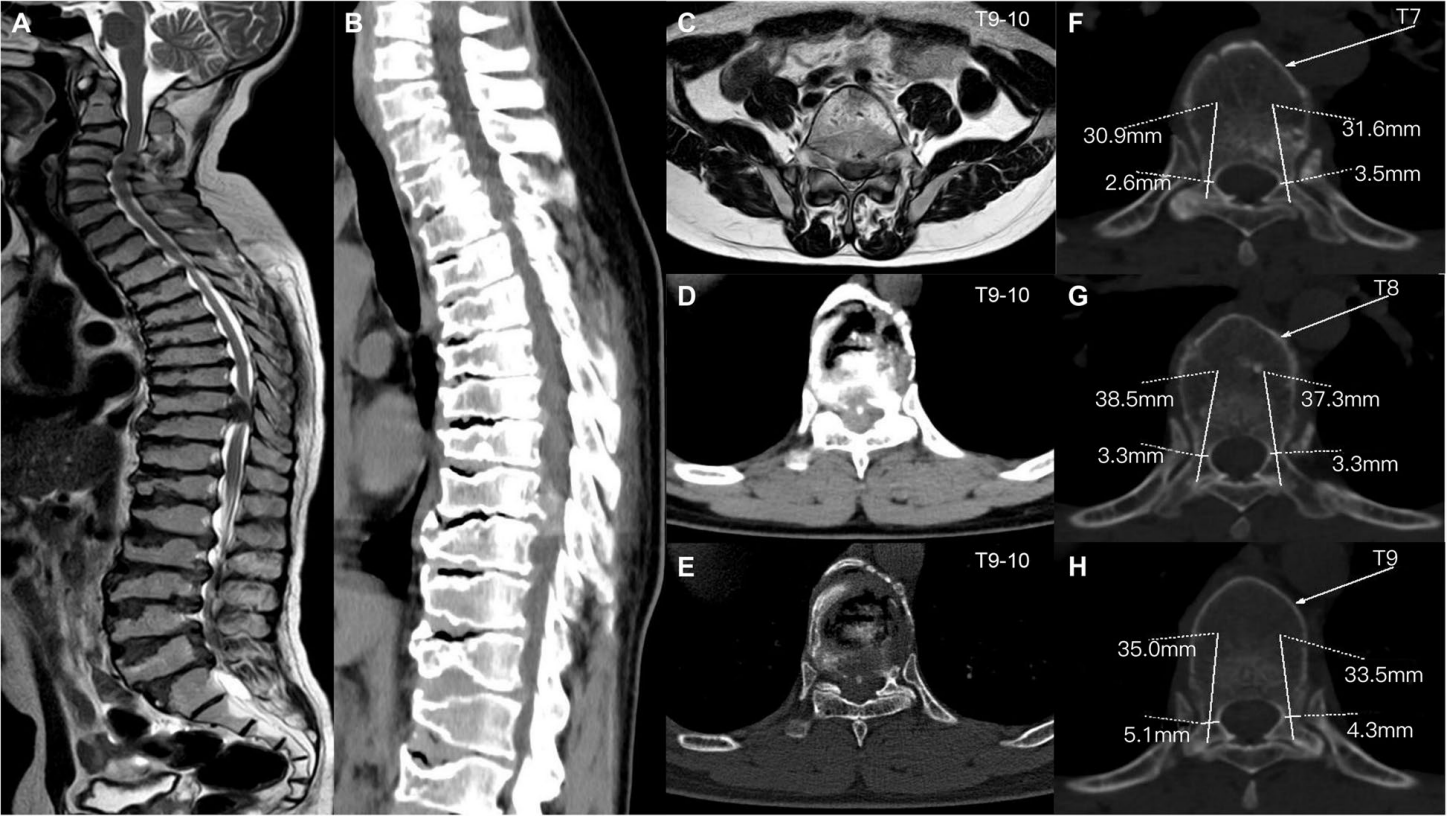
Preoperative T2-weighted magnetic resonance imaging (MRI) and computed tomography (CT) sagittal scan (a, b) showed multiple discs with reduced signal (intervertebral disc vacuum phenomenon) and spinal stenosis. Transverse view (c, d, e) of the intervertebral disc at T9–10 suggested posterior inferior disc prolapse with spinal cord compression. Measurements on a CT transverse view (f, g, h) of the pedicle suggested a small pedicle
